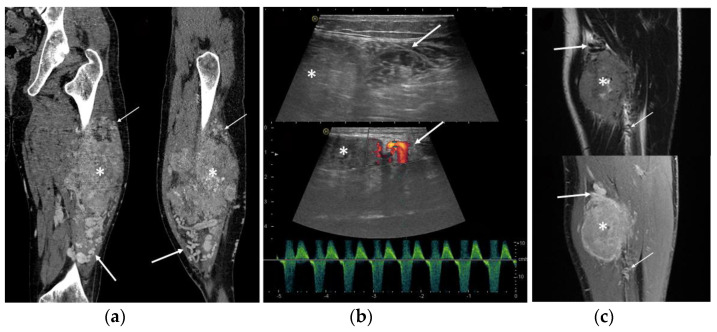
ASPS feeding vessels in different imaging modalities. Note the peritumoral tortuous vessels (arrows) proximally to the neoplasm (asterisk). (a) Coronal and sagittal view of CT scan; (b) ultrasound B-mode greyscale and color-Doppler with arterial pattern in the feeding vessels; (c) coronal views of T2-WI and contrast enhanced fat-saturated T1-WI.
